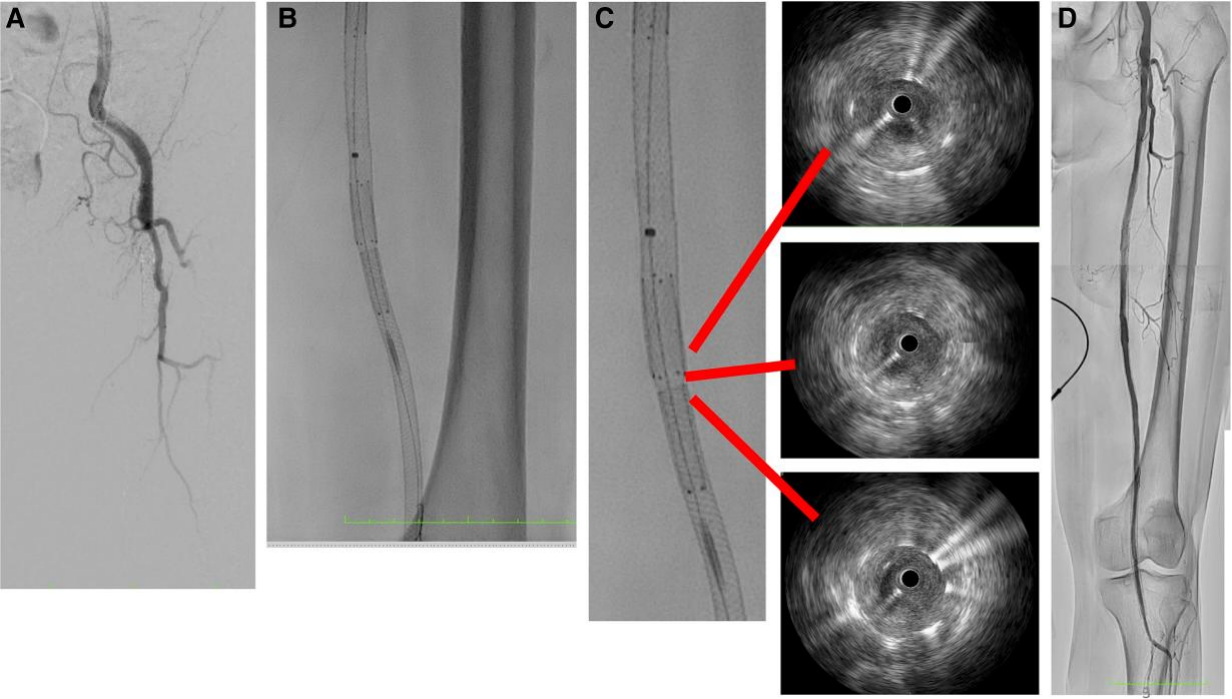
The initial angiogram revealed total occlusion of the superficial femoral artery ostium (*A*). In the gap, paclitaxel-eluting stent was implanted (*B*). Intravascular ultrasound showed good expansion and apposition of the overlap and gap lesion (*C*). The final result was a significant improvement in blood flow (*D*).
